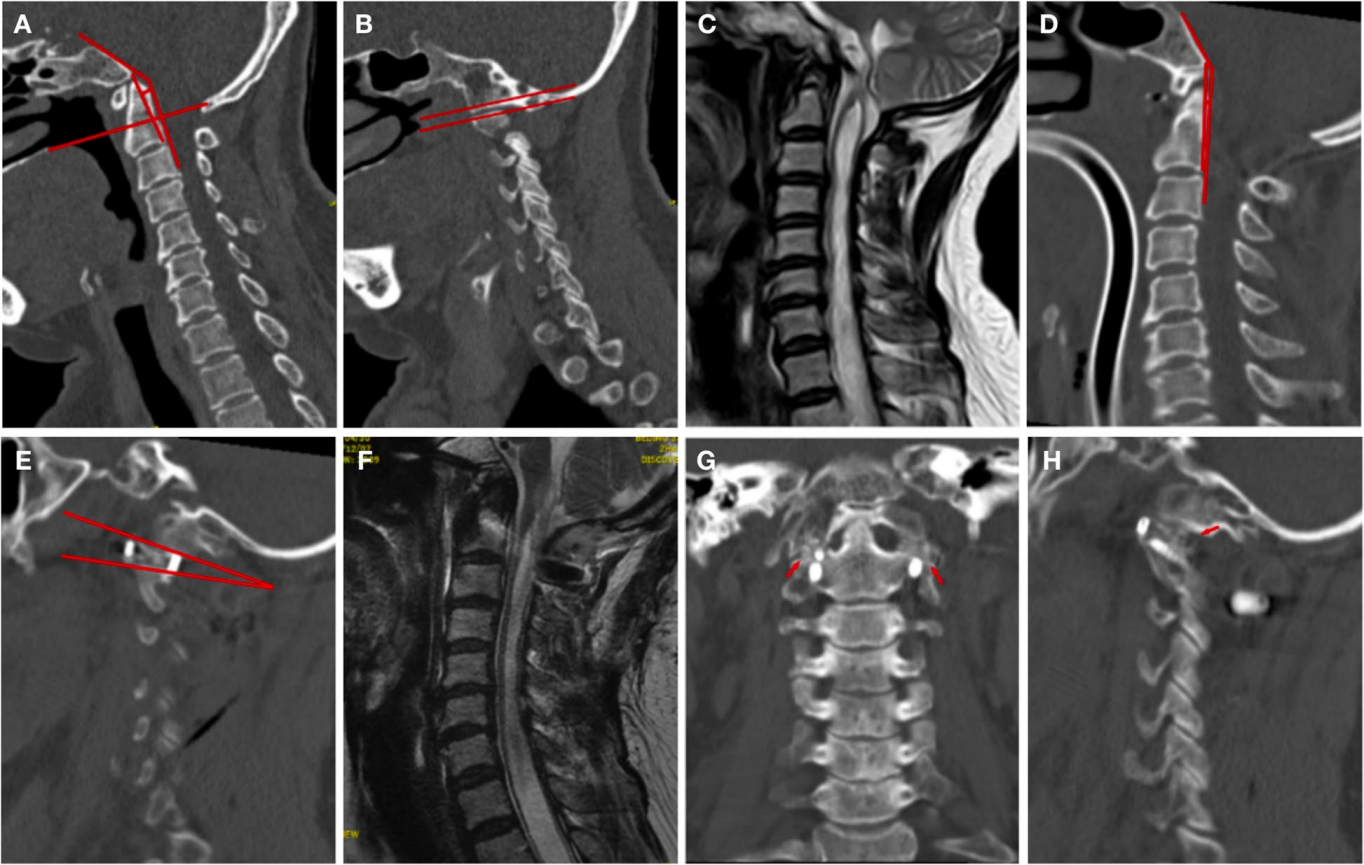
A 40-year-old woman presented with sensory disturbance, neck pain, and right-hand muscle atrophy for 15 months. After the operation, the patient’s sensory disturbance and neck pain were significantly relieved, and the Japanese Orthopedic Association (JOA) score increased from 13 to 15. A: Sagittal reconstructed computed tomography (CT) scan shows the presence of occipitalization of C1, BI without atlantoaxial dislocation (AAD), clivus-canal angle (CCA) = 143.1°, pB-C2 = 4.5 mm, and that the two facets of the C1–C2 joint were parallel (red lines) B. C: Preoperative magnetic resonance imaging (MRI) scan showing associated Chiari malformation, and syringomyelia. D: Postoperative sagittal CT showed that the odontoid process was lowered and the CCA increased, CCA = 152.4° and pB-C2 = 1.9 mm. The two facets of the C1–C2 joint are not parallel; the C1 joint rotates upward and backward E. F: Postoperative sagittal MRI showed a slight decrease in syringomyelia. G and H: The 12-month CT showed that bony fusion had occurred between the C1–C2 articular surfaces
